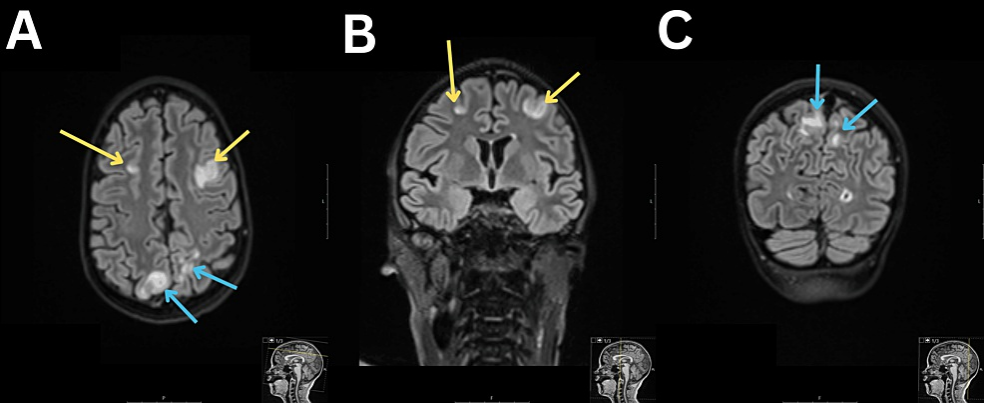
T2-Weighted MRI MRI FLAIR images showing hyperintense lesions in cortical and subcortical white matter in the frontal (A and B, yellow arrows) and parietal regions (A and C, blue arrows). MRI, magnetic resonance imaging; FLAIR, fluid-attenuated inversion recovery.
